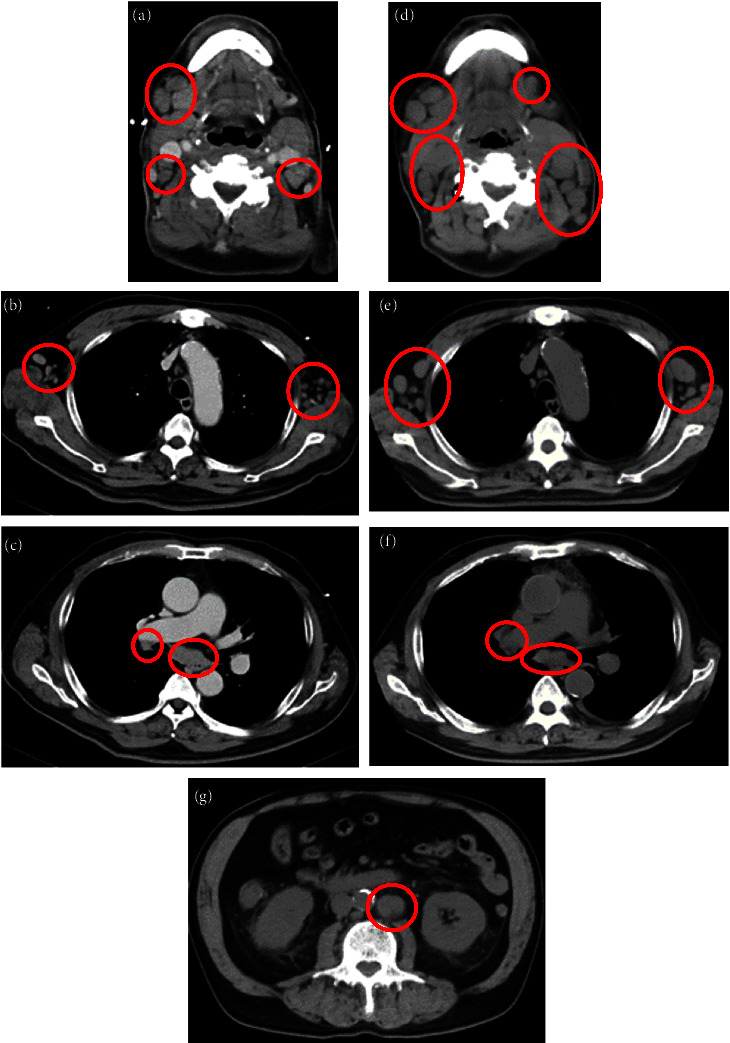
Computed tomography revealed multiple lymph node swellings. Computed tomography scans at the first visit (a, b, and c) and four months later (d, e, f, and g), shows the cervical lymph nodes (a, d), axillary lymph nodes (b, e), mediastinal lymph nodes (c, f), and para-aortic lymph node (g).
